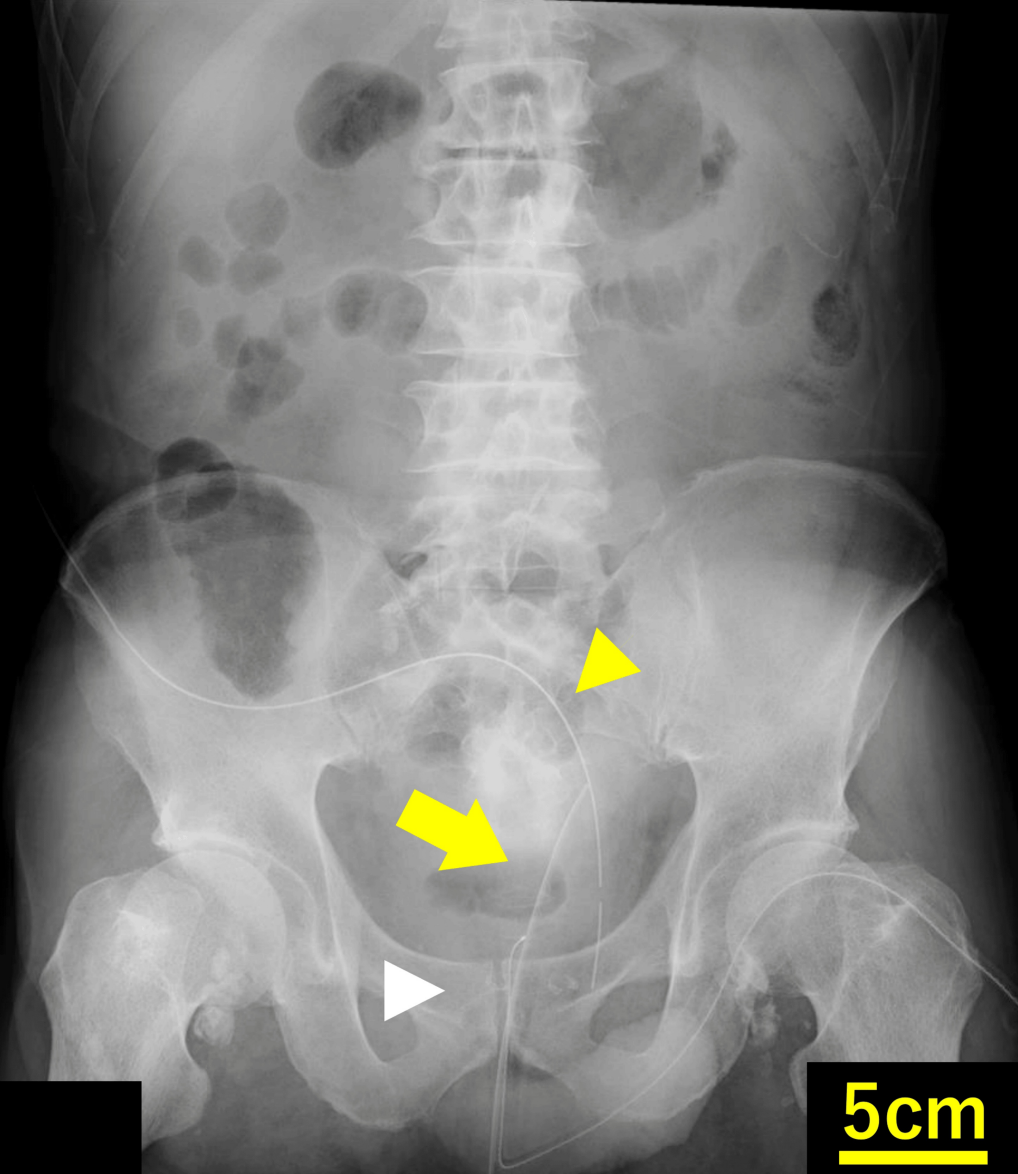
Abdominal radiograph on POD 1 showing the transanal drainage tube (yellow arrow), the anastomotic drain (yellow arrowhead), and the anastomotic site (white arrowhead).
